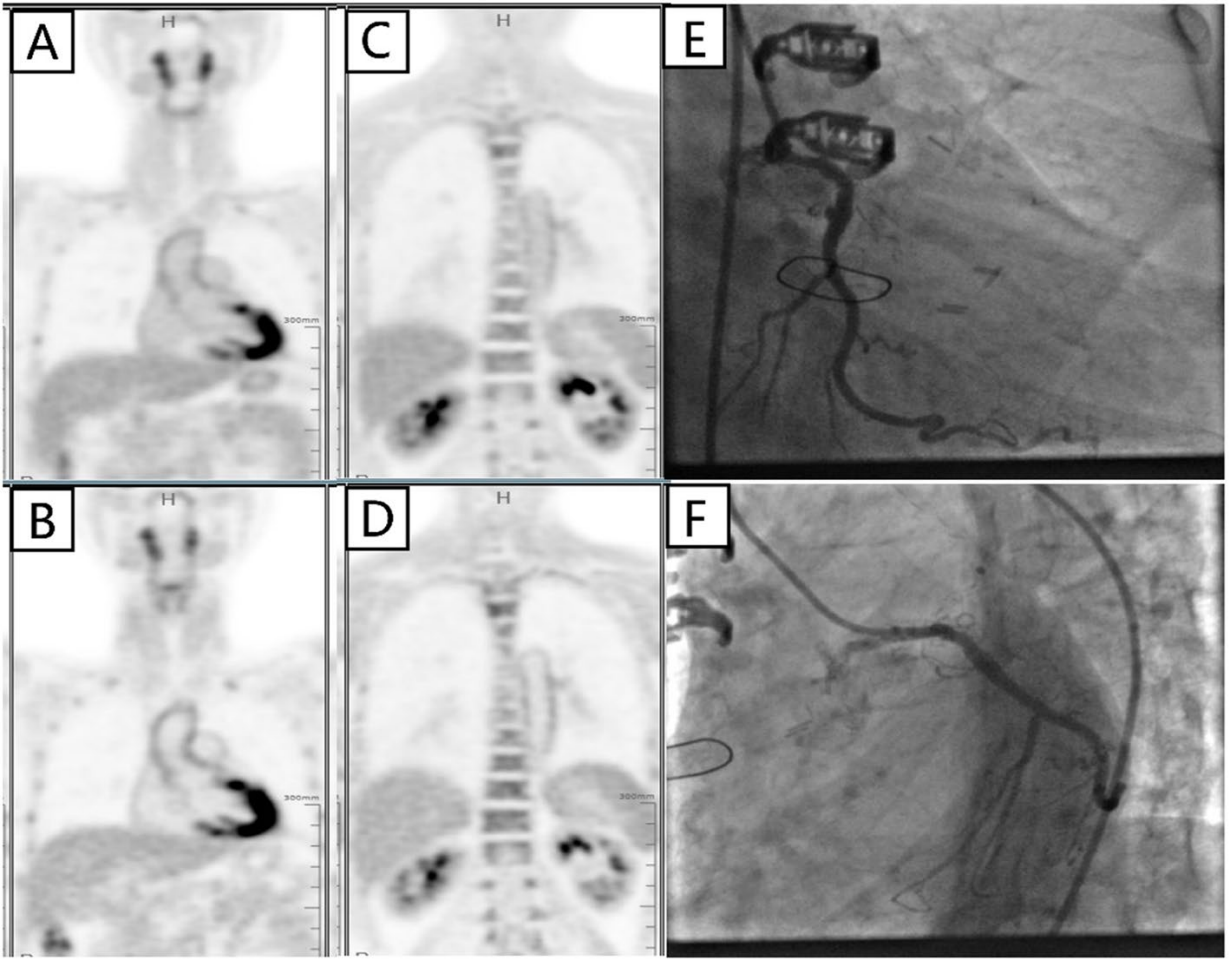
Positron emission tomography computed tomography on February 15, 2022, and coronary angiography on August 15, 2022. (A) and (B) represented images of ascending aorta after 2-[fluorine-18]-fluoro-2-deoxy-D-glucose injection 2 h and 4 h, respectively; (C) and (D) stands for descending aorta after 2-[fluorine-18]-fluoro-2-deoxy-D-glucose injection 2 h and 4 h, respectively; (E) and (F) Follow up angiogram showed well patency of left circumflex coronary artery stent
